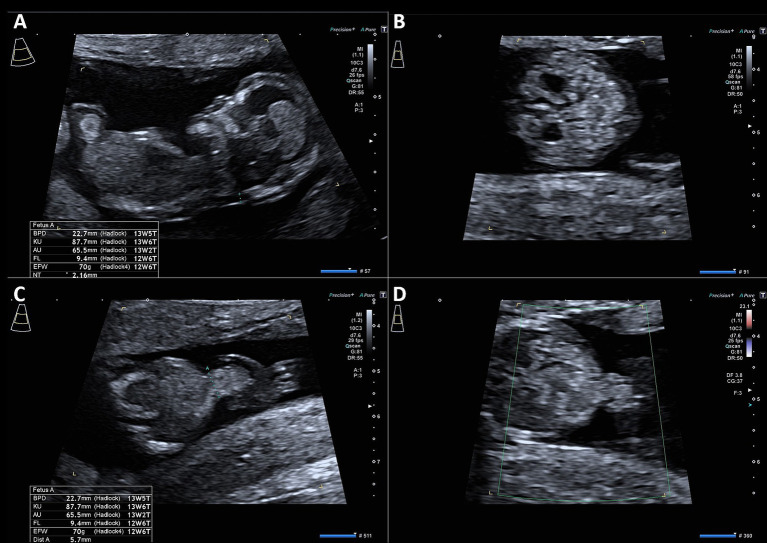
Ultrasound images of first-trimester screening showing the measurement of nuchal translucency (A), bilateral hydronephrosis (B), and omphalocele (C,D).
